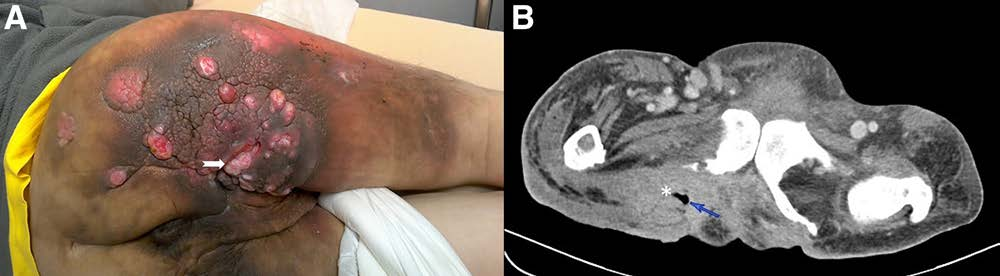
Initial presentation of a 45-year-old man with right buttock and thigh necrotizing soft tissue infection. (A) Clinical picture showing thickened skin with hyperpigmentation and multiple discharging sinuses. An ischial pressure ulcer at the center. (arrow) (B) Contrast-enhanced computed tomography image showing the soft tissue mass (star sign) with free air (arrow).
